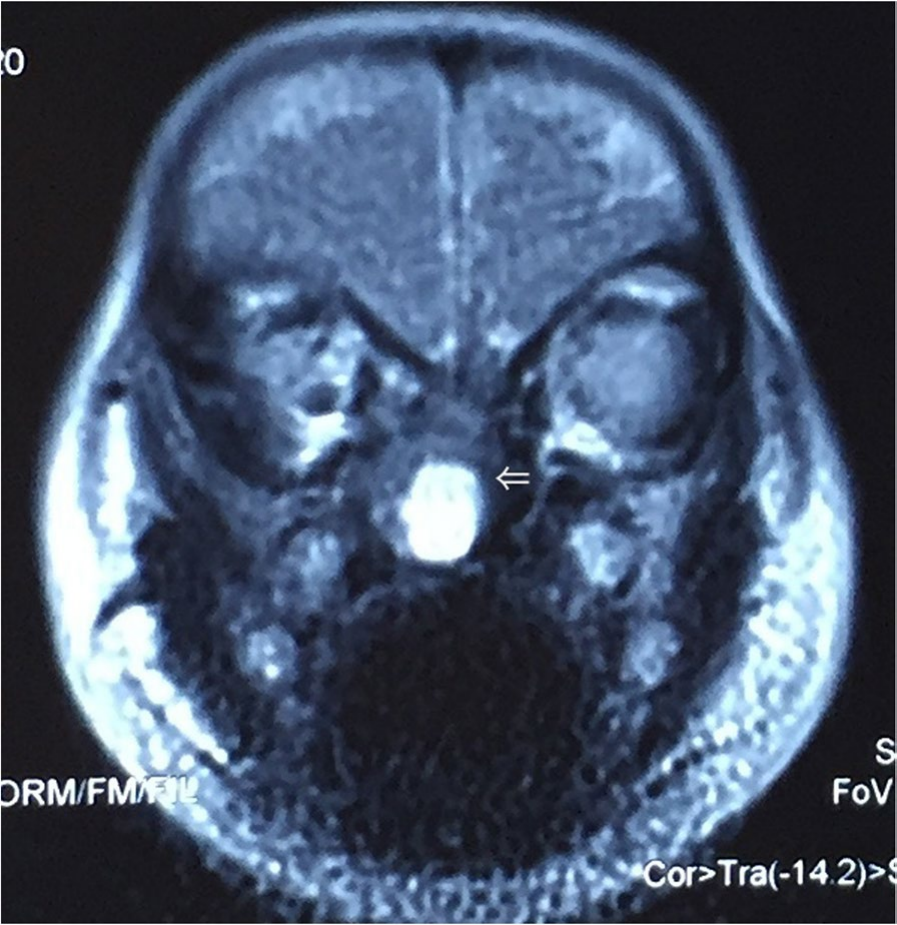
MRI Coronal image revealed right well-capsulated intranasal mass with T2-weighted hyperintense myxoid and cystic components
